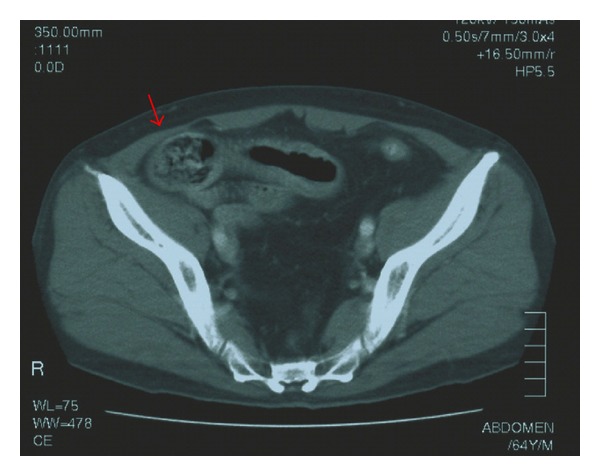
Pelvic CT shows a dilated small bowel loop and a mass measuring 4 cm.
